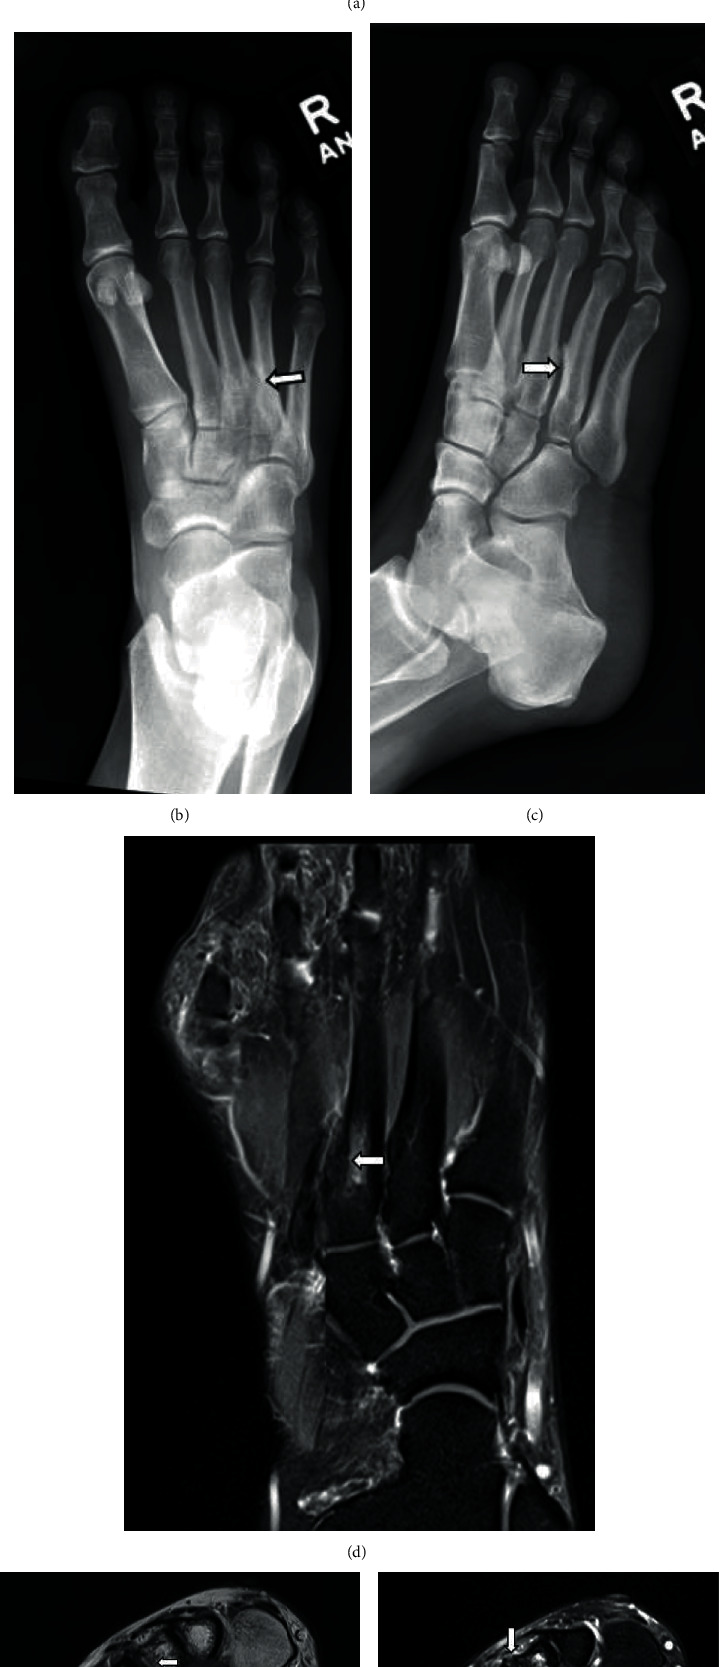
29-year-old female with dorsolateral foot pain. Ultrasound in axial view of the proximal metatarsals (a) shows abnormal bony overgrowth in the dorsal border of the third and fourth intermetatarsal space. This is associated with adventitial bursal formation, soft tissue oedema, and increased vascularity. AP (b) and oblique (c) radiographs show abnormal articulation at the base of the third and fourth metatarsals with sclerosis at the site of the approximation. Coronal PDFS (d) with nonosseous coalition at the third and fourth intermetatarsal spaces. Associated periostitis and intramedullary oedema signal. Axial PD (e) and PDFS (f). Marrow oedema signal. Osteitis and periostitis. Abnormal articulation at this level with surrounding bone spurring.
